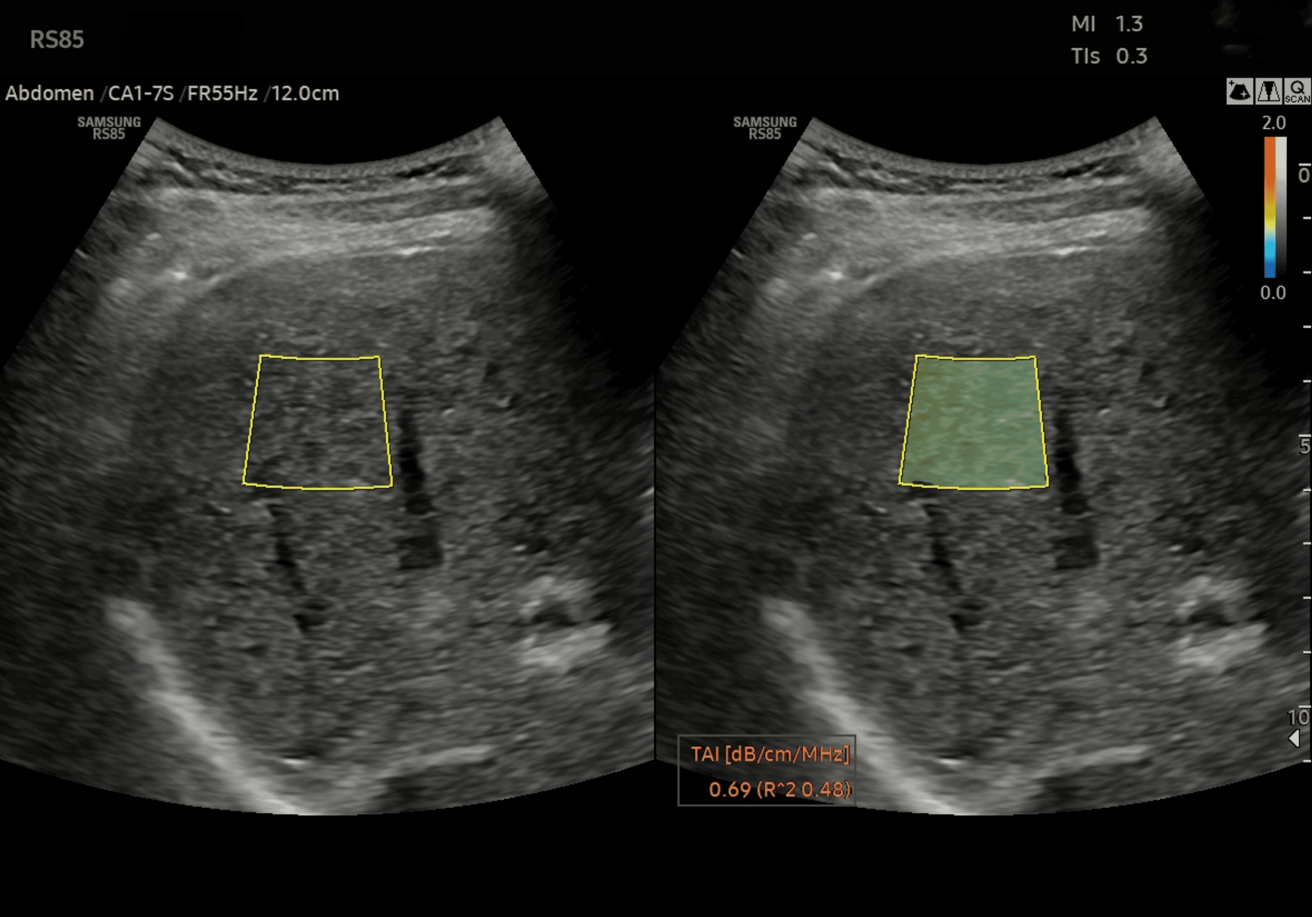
Representative anonymized clinical report generated by the Samsung RS85 Prestige ultrasound system, demonstrating TAI measurement and visual region of interest placement for quantitative assessment of hepatic steatosis. All personal identifiers have been removed. TAI = tissue attenuation imaging
